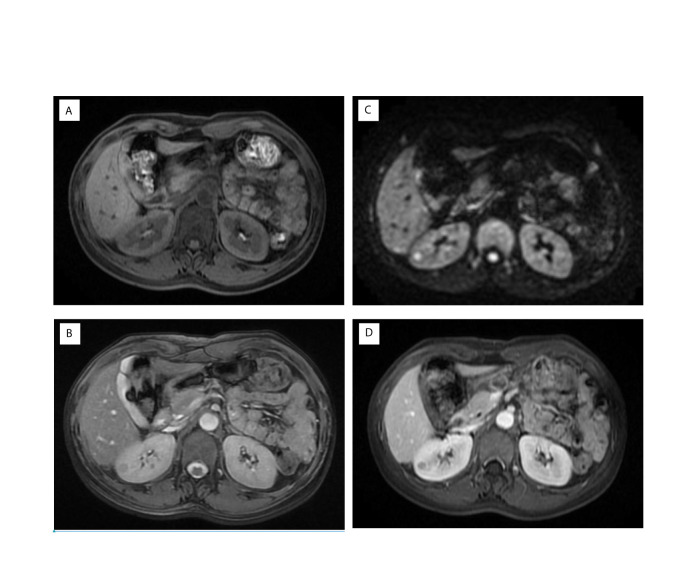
Magnetic resonance imaging (MRI) images of Patient No. 8. Signals of (A) T1, (B) T2, and (C) Diffusion-weighted images of non-contrast MRI. Signal of (D) arterial phase of contrast MRI.
